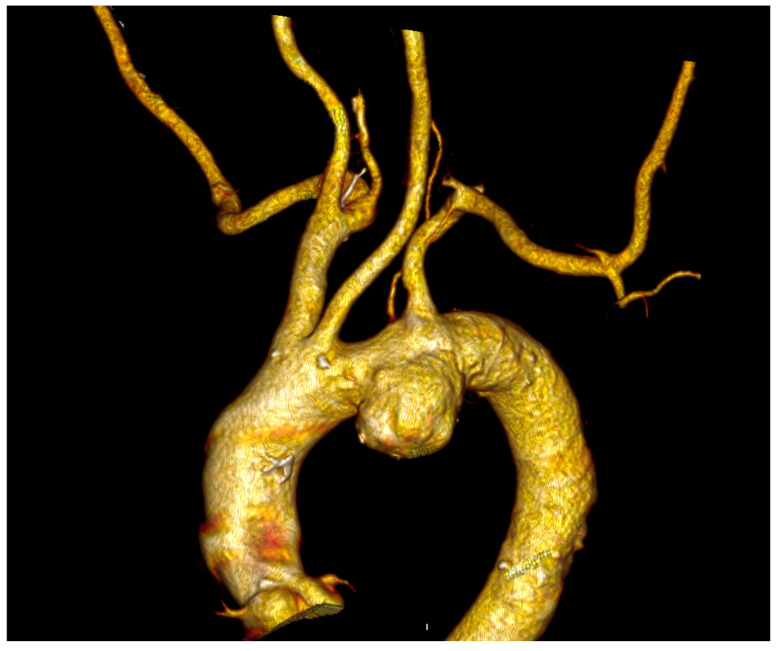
Preoperative Computed Tomography Angiography of a patient with a left vertebral artery arising directly from the aortic arch and affected with an aortic arch aneurysm treated at our institution.
